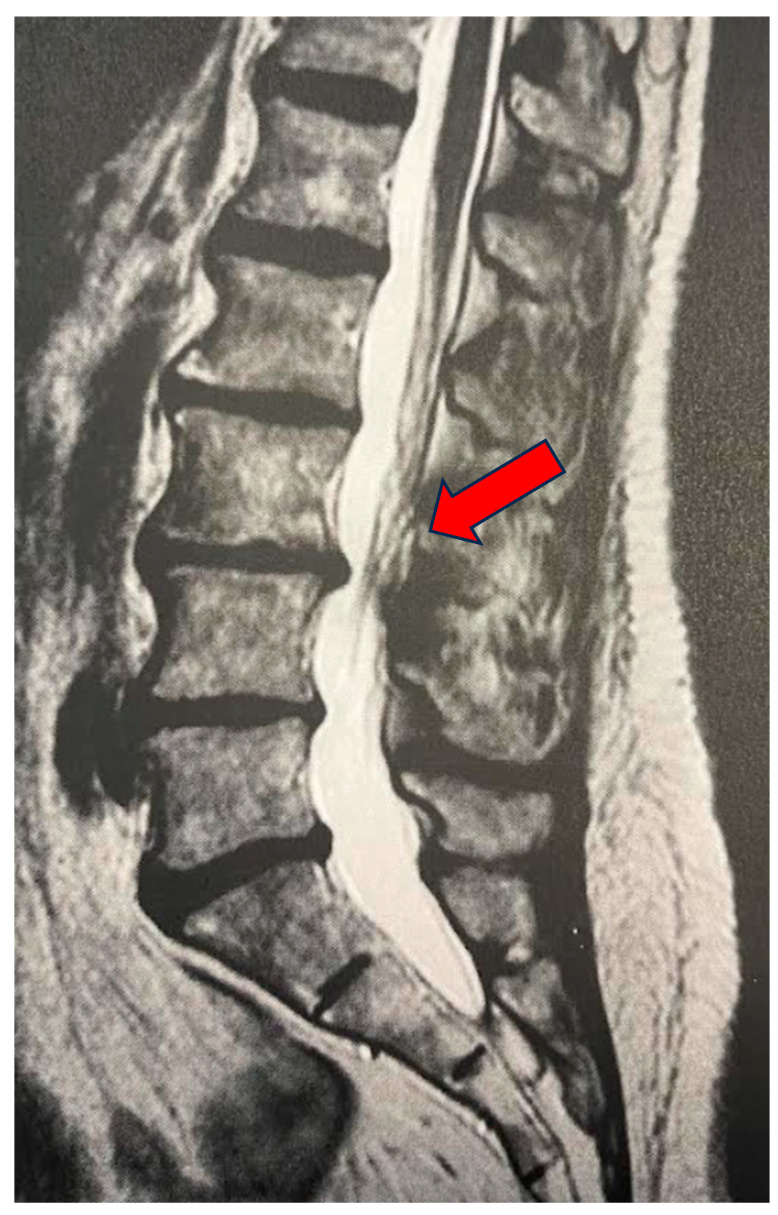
LPR of 78-year-old female. Sagittal MRI: median subligamentous disc herniation at L3–L4 (arrow). Complete resolution of low back pain symptoms at both the one-month and one-year follow-up after treatment.
